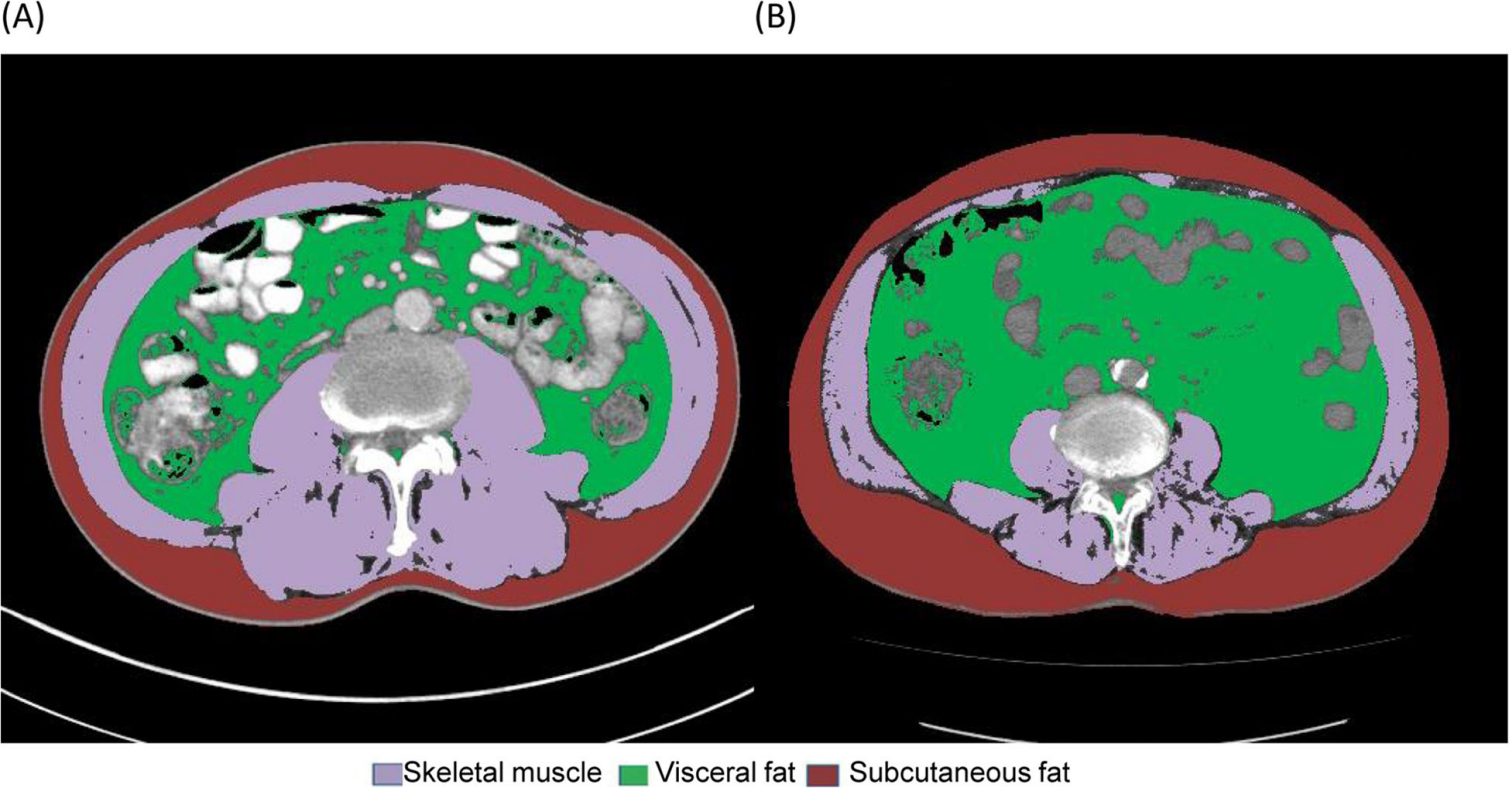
Cross-sectional computed tomography of two patients, body composition evaluated at the third lumbar vertebral level. Two patients with similar BMI values (24.4 vs. 24.8 kg/m^2^); one (A) had more skeletal muscle and less visceral adiposity than the other (B) (SMI, 63.7 vs.42.9 cm^2^/m^2^; VSR, 1.01 vs. 1.83). BMI, body mass index; SMI, skeletal muscle index; VSR, visceral-to-subcutaneous fat ratio
